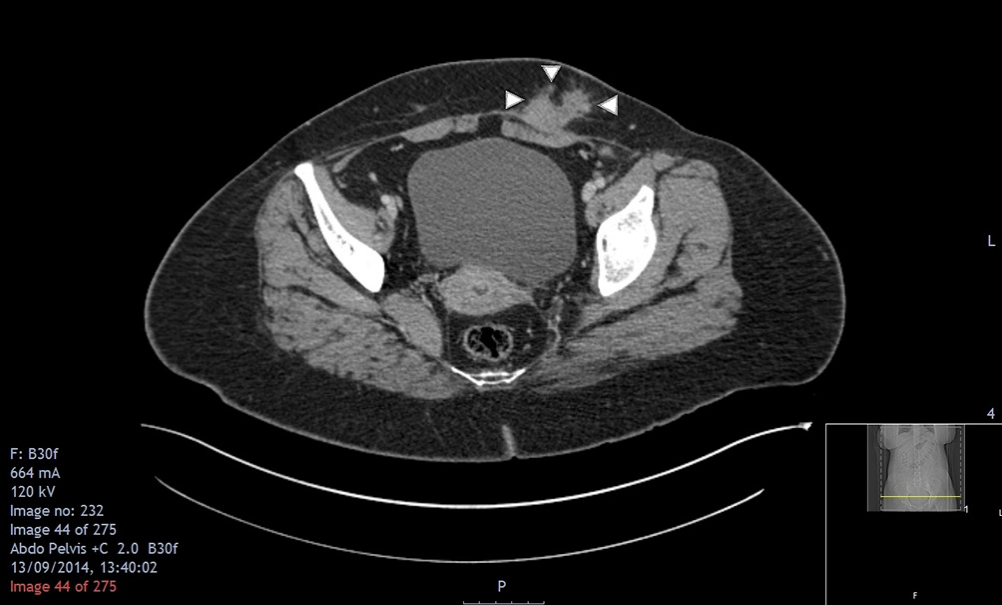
Axial contrasted CT image showing a well-defined soft tissue nodule with postcontrast enhancement in the soft tissue, and streakiness in the surrounding tissue, extending anteriorly from the rectus muscle.
